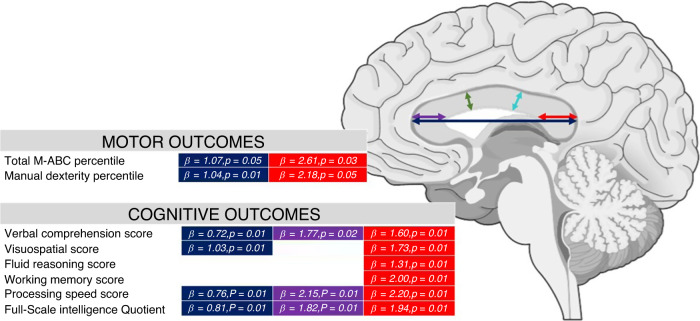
Linear CC measurements related with motor and cognitive subscales. All models were adjusted by GA at birth, sex and age at MRI. Only statistically significant results were expressed in the tables. CC corpus callosum, GA gestational age, MRI magnetic resonance imaging.
